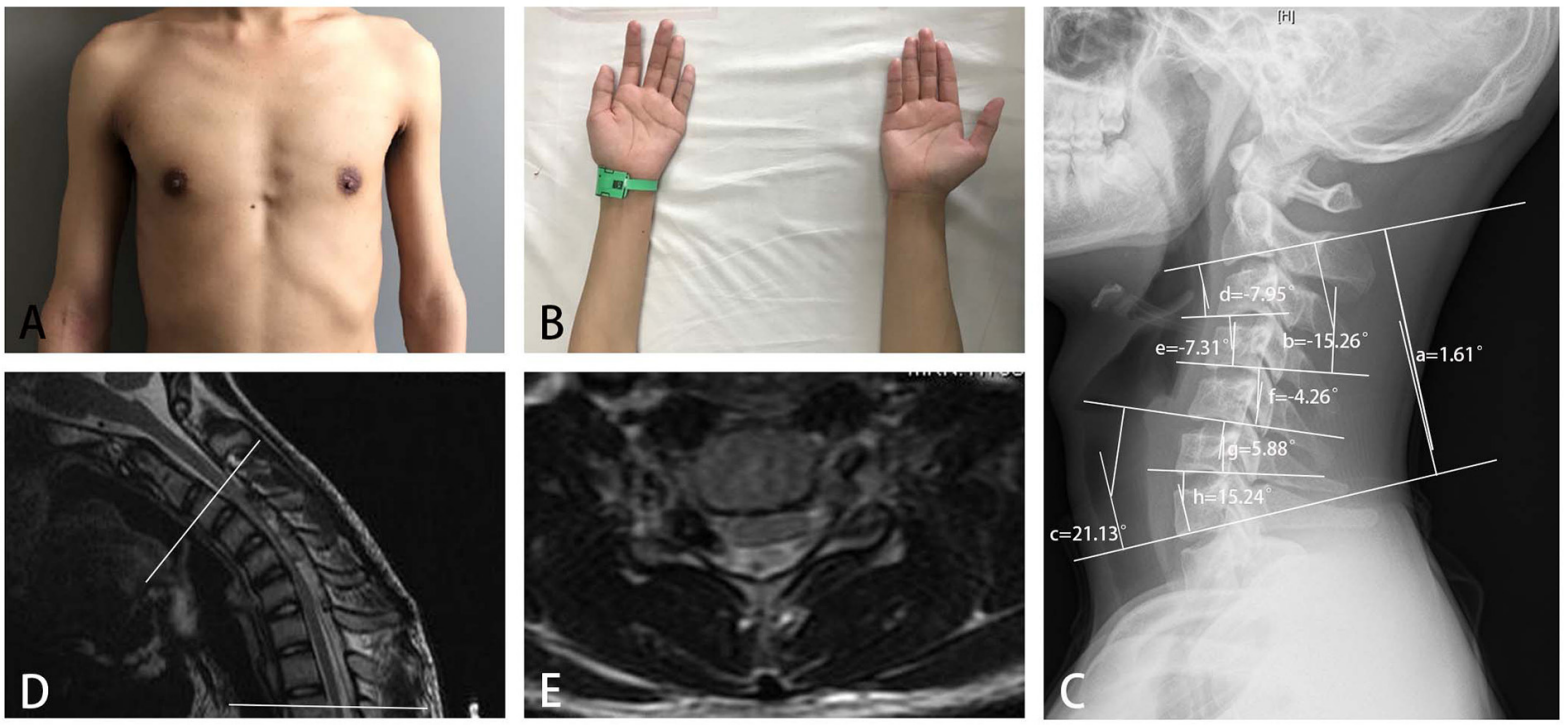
A 16-year-old boy with obvious weakness and atrophy of muscles of deltoid and biceps for 3 months (A), and slight difficulty in finger extension (B), was diagnosed with HD with proximal involvement. A neurogenic injury from C5 to C8 was found in electromyography. (C) An abnormal cervical curvature in the upper part of the cervical spine, (a) C2-7 Cobb angle = 1.61 degrees; (b) C2-4 Cobb angle = −15.26 degrees; (c) C5-7 Cobb angle = 21.13 degrees; (d) C2-3 Cobb angle = −7.95 degrees; (e) C3-4 Cobb angle = −7.31 degrees; (f) C4-5 Cobb angle = −4.26 degrees; (g) C5-6 Cobb angle = 5.88 degrees; and (h) C6-7 Cobb angle = 15.24 degrees; loss of attachment was found in cervical-flexion MRI in seven segments from C3 to T2 (between the two white lines), (D) sagittal plane, and (E) cross-sectional plane.
